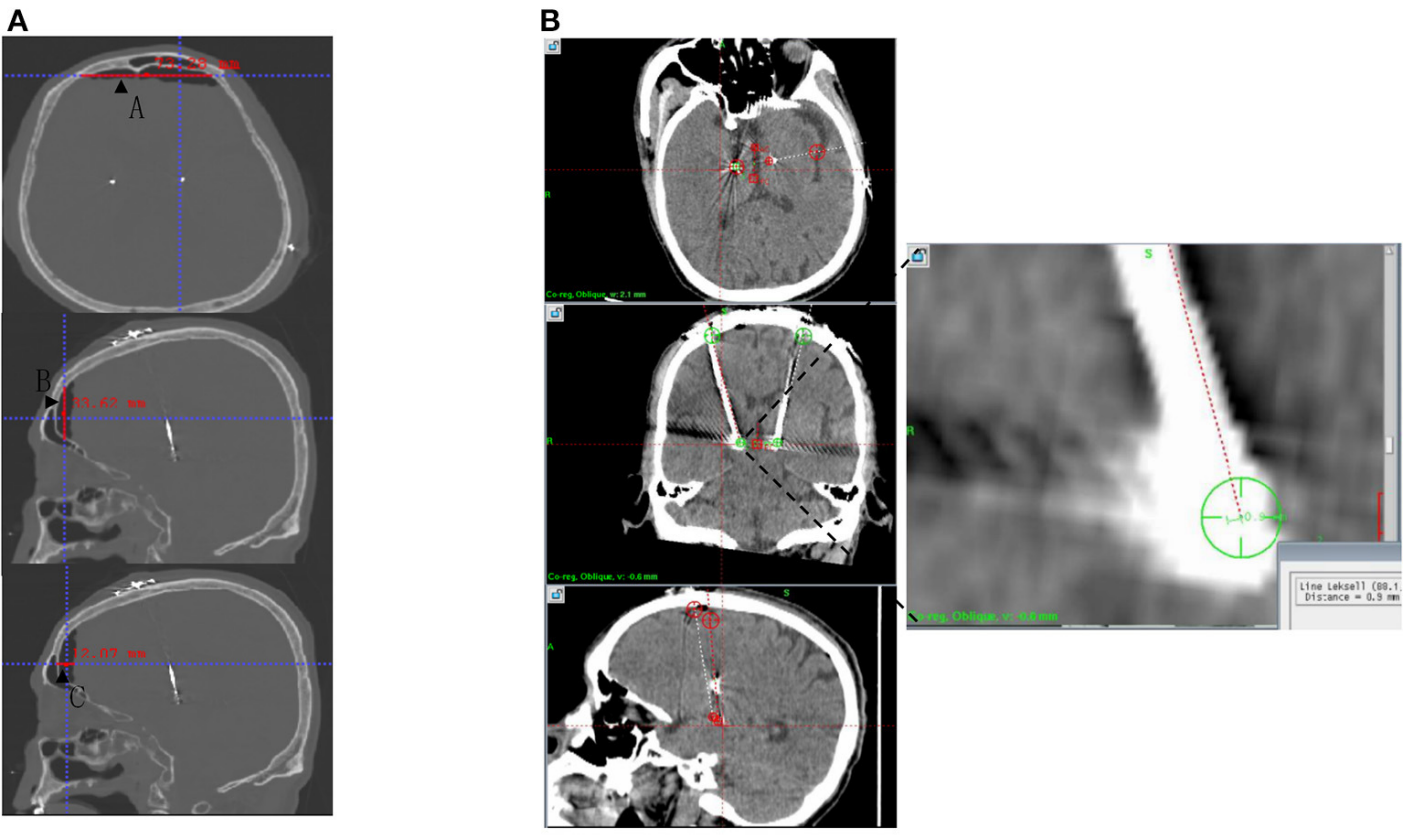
Postoperative CT data measurement. (A) PPV and CBS were calculated from A, B, and C under the same coordinate system measured after postoperative CT postprocessing. (B) Postoperative CT was fused with preoperative planning to measure EFE. CBS, Cortical brain shift; CT, computed tomography; EFE, Electrode fusion error; PPV, Postoperative pneumocephalus volume.
